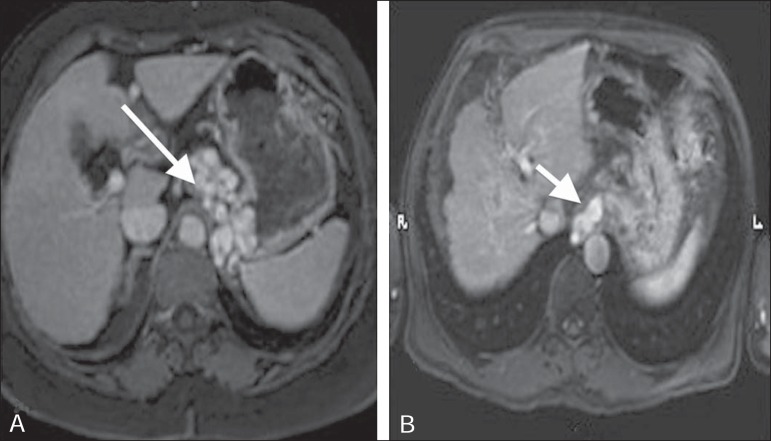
A,B: Contrast-enhanced, T1-weighted magnetic resonance
